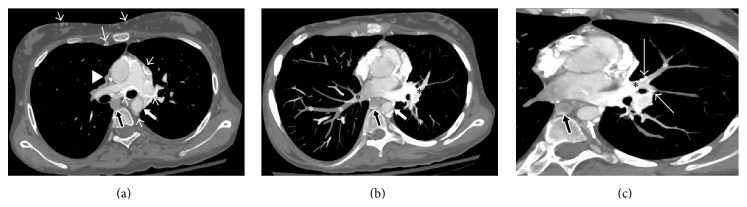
Enhanced axial chest CT image (a) and oblique axial thin-slab maximum-intensity-projection (MIP) images (b, c) inferior to (a) show thrombosed and stenosed SVC (arrowhead) and distended and occluded azygos vein (black block arrow). There are chest wall, accessory hemiazygos, and paravertebral, intense mediastinal venous collaterals (short arrows). Central superior pulmonary veins (black asterisks) are opacified through adjacent collateral (long white arrows) as compared to nonopacified peripheral portions (white asterisks). Note the contrast filled aorta (white block arrow).
